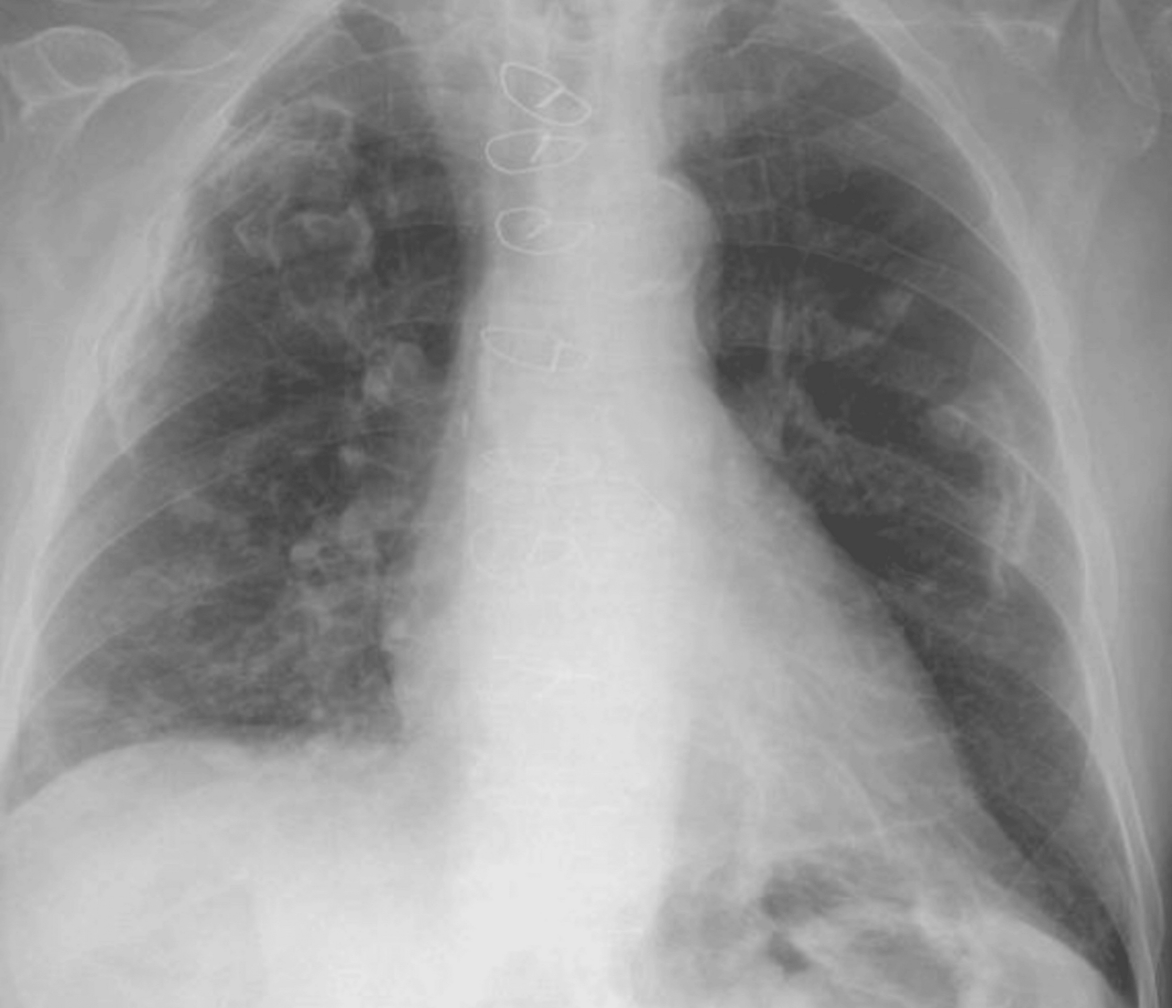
Chest X-ray Bilateral pleural plaques were noted. Sternotomy wires and aortic valve replacement were noted. There appears to be a new focus of consolidation in the right upper zone, medial to the large pleural plaque. The film is, however, significantly rotated, and this appearance may be due to a difference in the projection of the plaque. Old clavicle fractures were noted.
